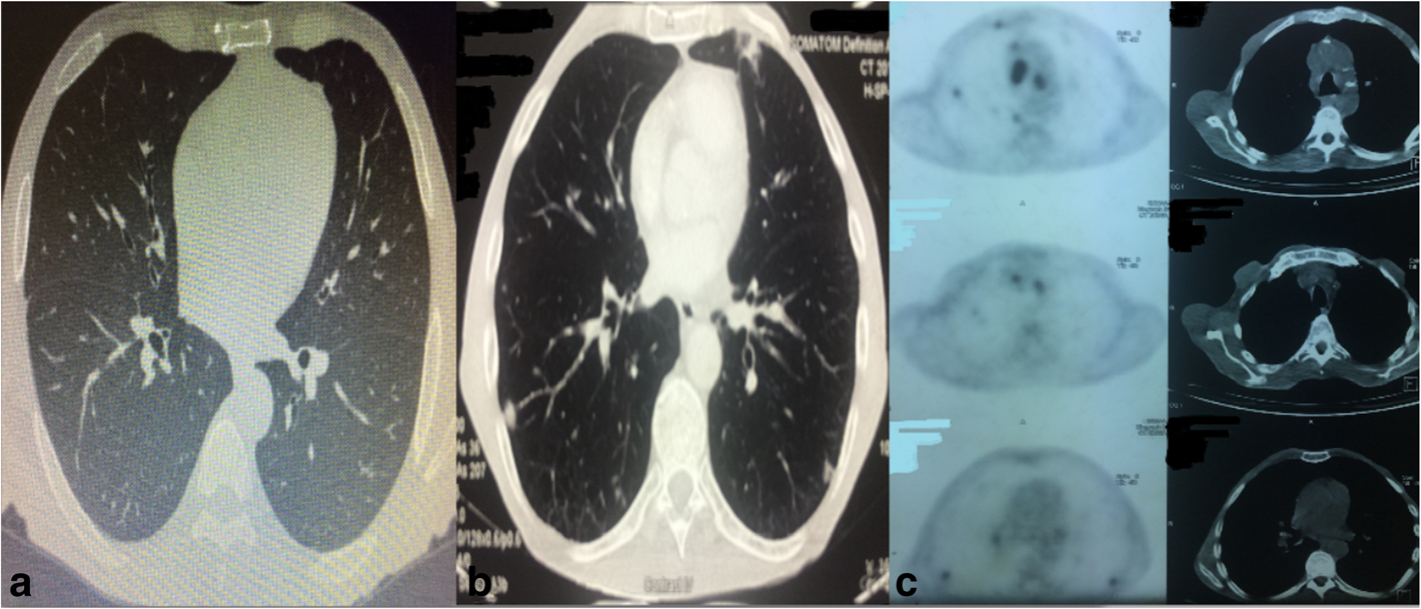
a Preoperative CT thorax showing the absence of metastasis. b Postoperative CT thorax with metastatic lung lesions. c PET-CT confirming the diagnosis of metastatic lung lesions
